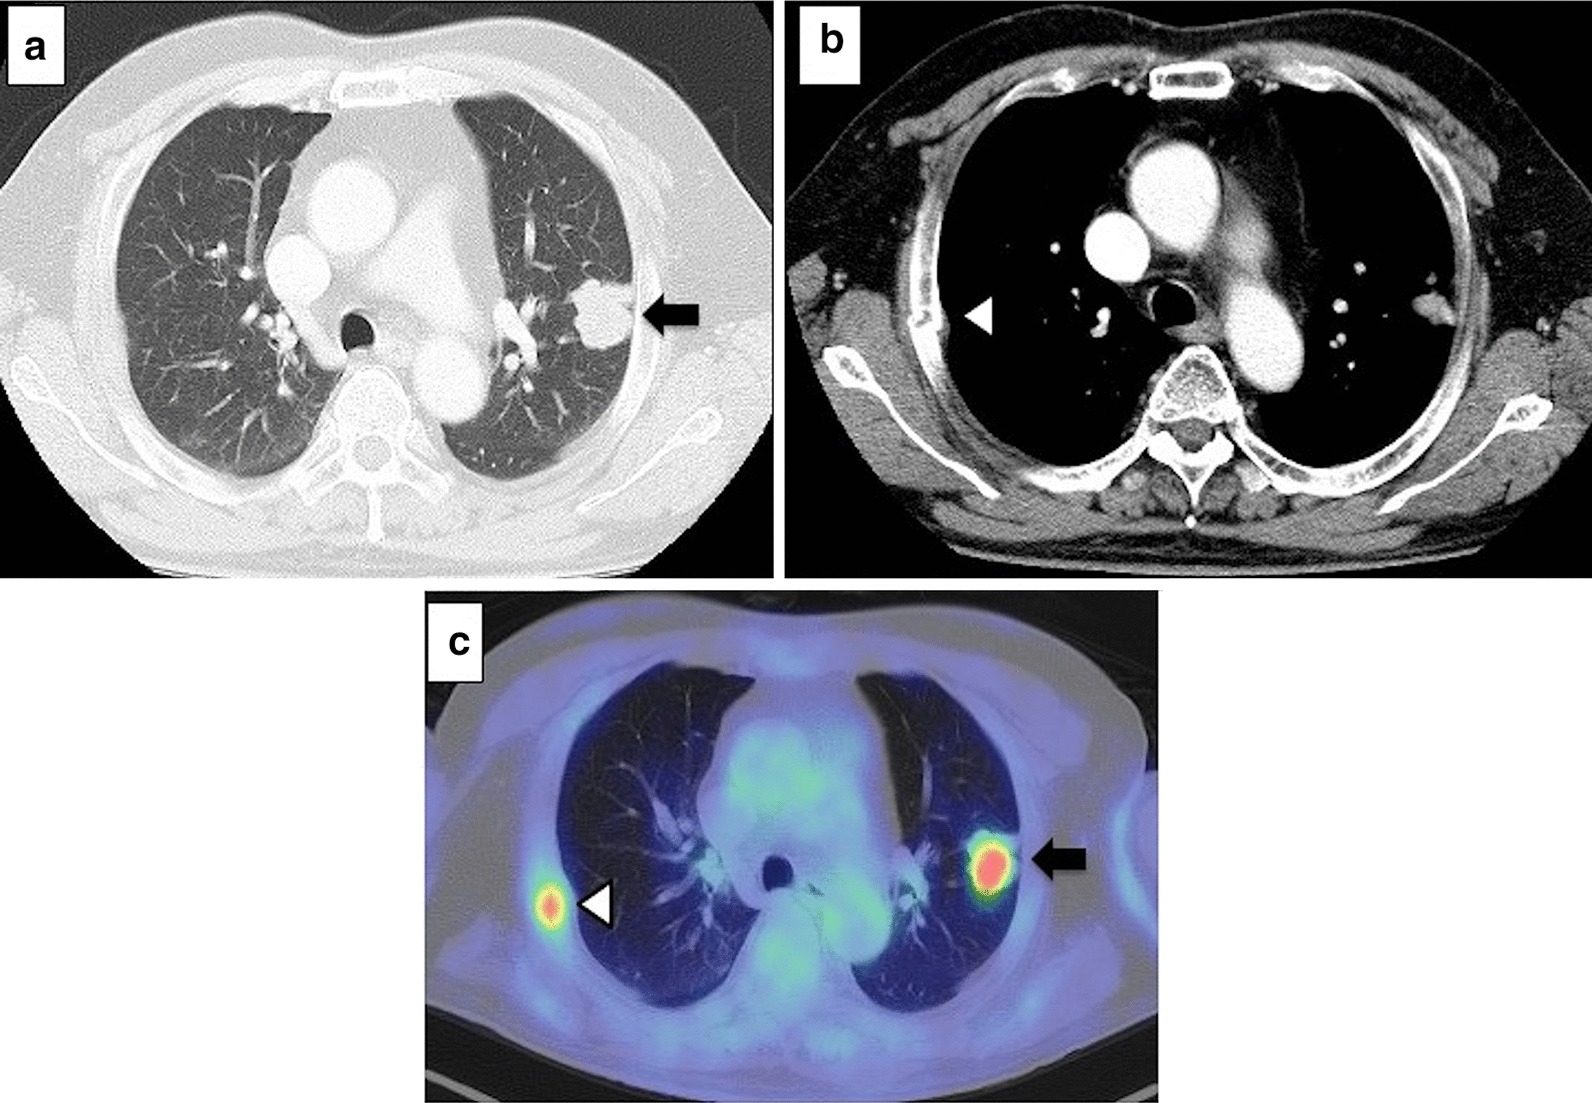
a, b Chest computed tomography shows a mass located in left upper lobe (←) and no pulmonary bullae. Fractures of the right third, fourth, and fifth ribs were noted (white left-pointing triangle). c Fluorine-18-fluorodeoxyglucose positron emission tomography/computed tomography demonstrated uptakes in the tumor of the left upper lobe (←) and the site of rib fractures
